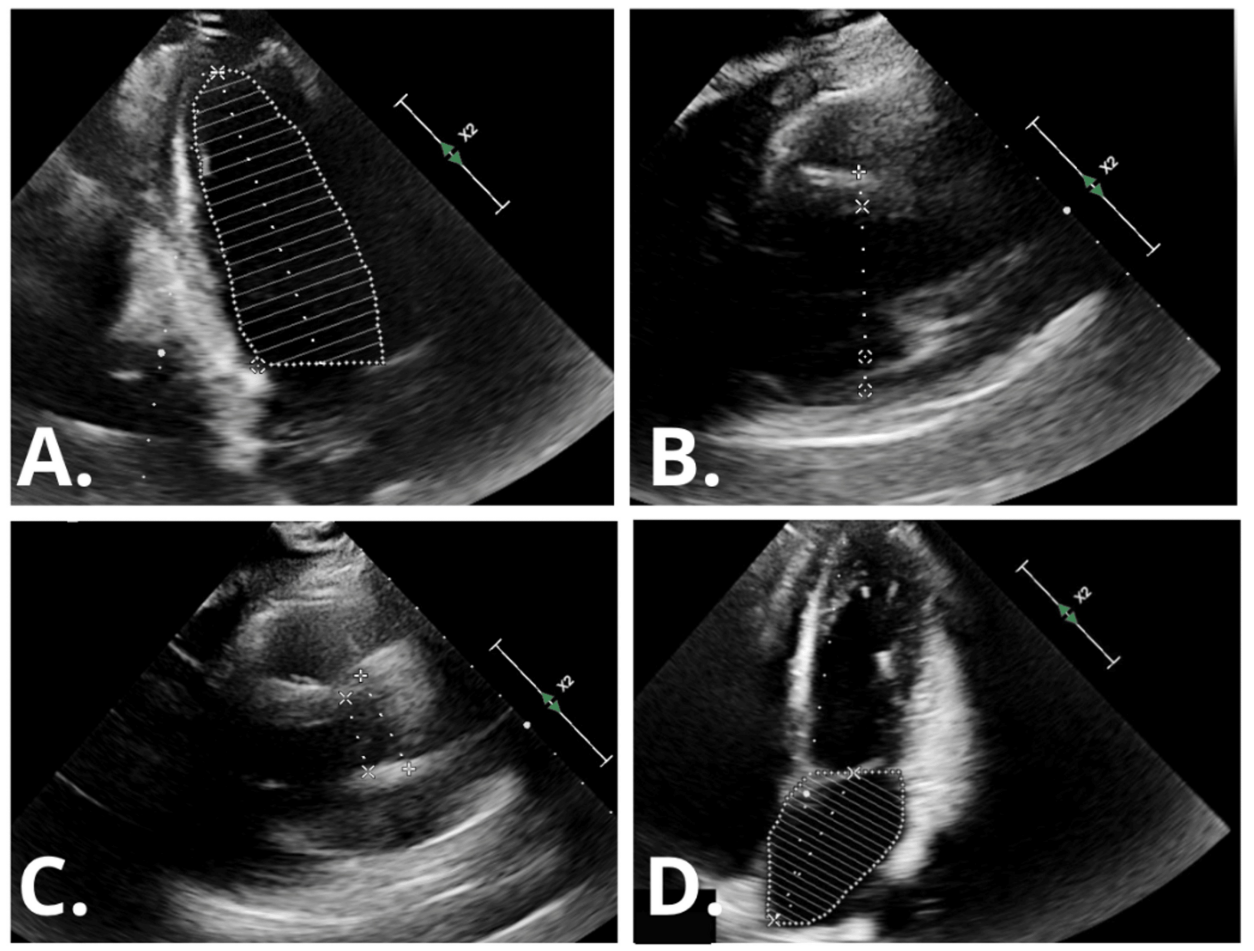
Transthoracic echocardiography findings (A) Apical two-chamber view with endocardial tracing of the left ventricle for volumetric assessment using Simpson’s method. (B) Apical view demonstrating measurement of left ventricular diameter. (C) View showing measurement of the LVOT and aortic root. (D) Apical four-chamber view demonstrating left atrial planimetry. LVOT, left ventricular outflow tract
